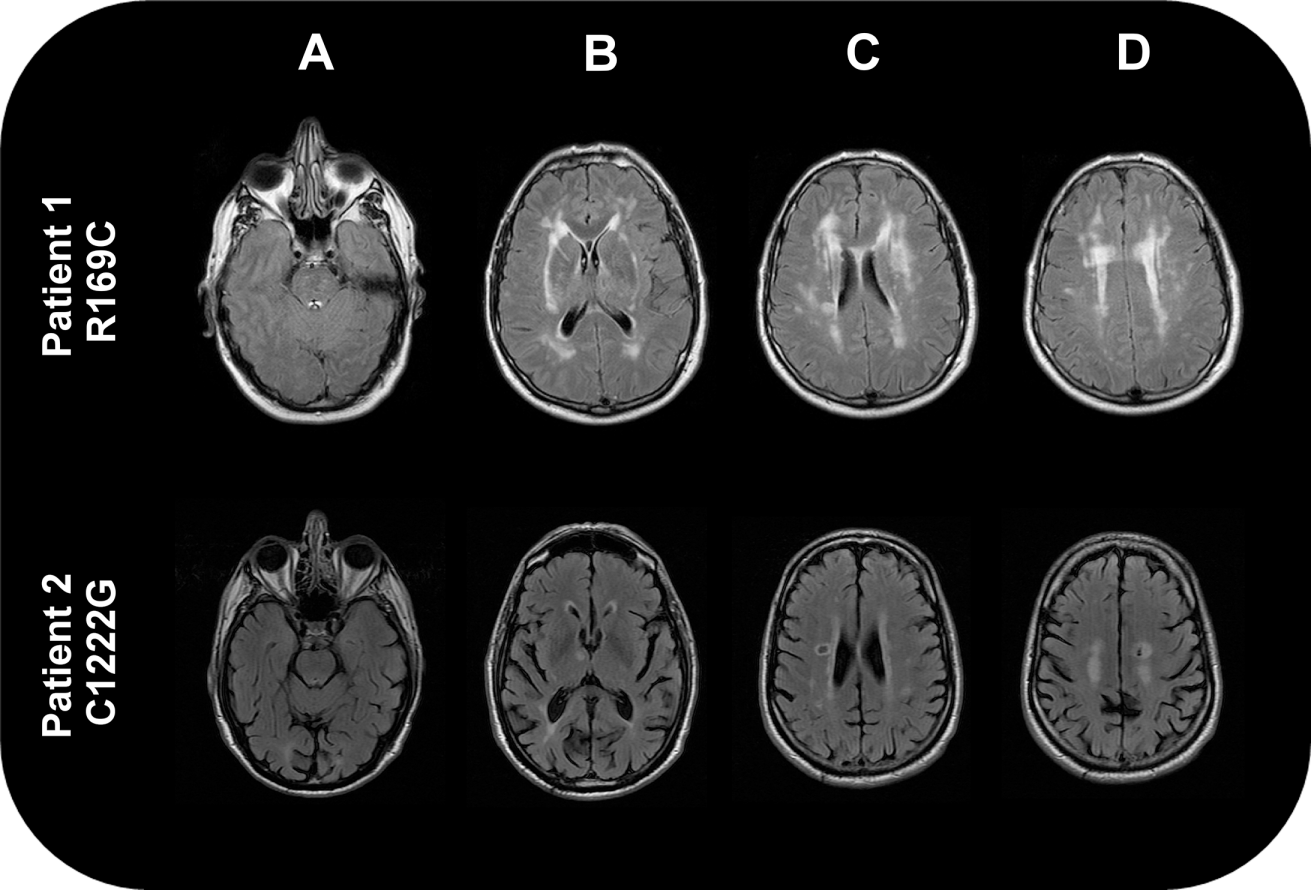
FLAIR Brain MRIs scans from two of the patients with notch 3 mutations. The upper panel 1 shows images from patient 1 (R169C), and the lower panel two shows images from patient 2 panel 2 (C1222G). These show leukoaraiosis in both cases (images C and D) but minimal anterior pole involvement in patient 1 (image upper panel A) and no involvement in patient 2 (image lower panel A). Patient 1 also shows confluent external capsule involvement (image B), but this is absent from patient 4 (lower panel B).
